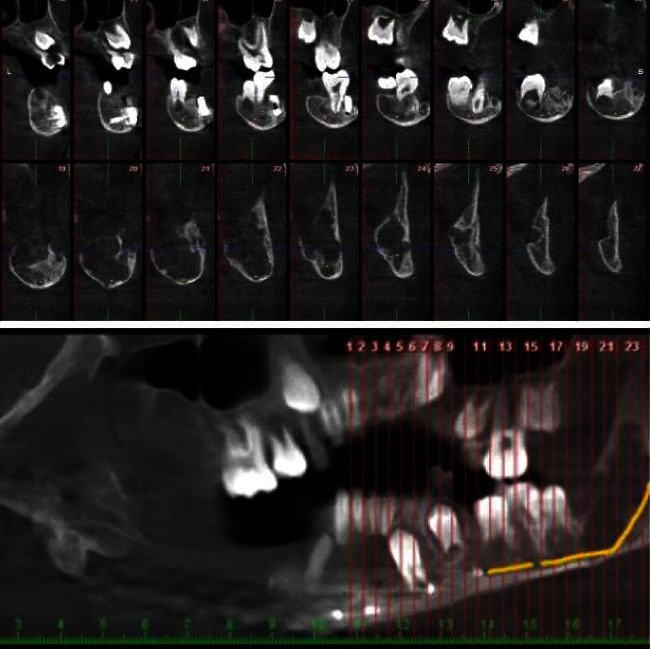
The cross-sectional images in the CBCT scan.
